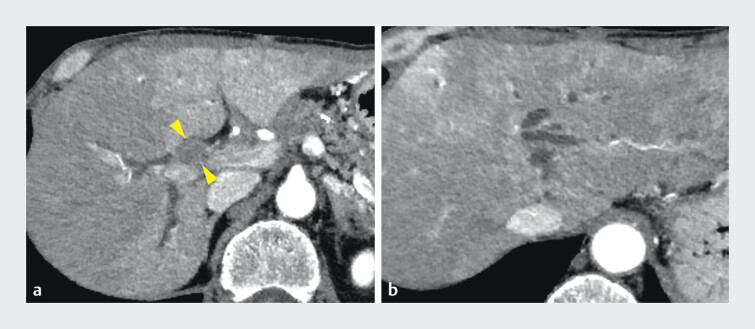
Contrast-enhanced computed tomography (CT) shows a hypovascular tumor at the hepatic hilum (arrowheads) and dilated bilateral intrahepatic bile ducts.
